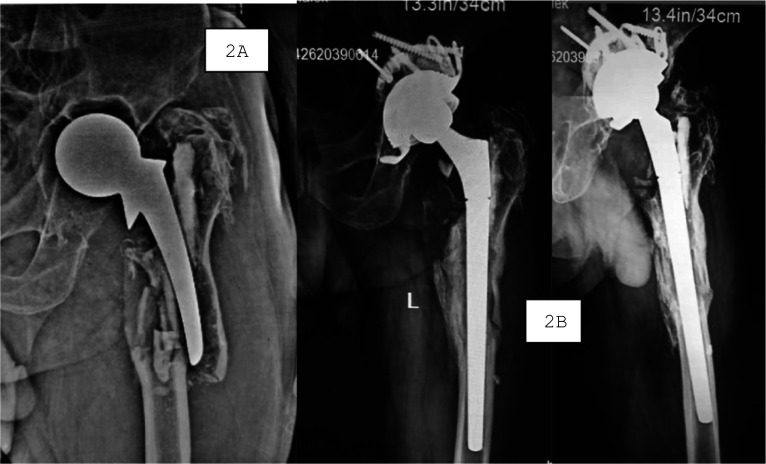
A 62-year-old male patient who underwent revision total hip arthroplasty using a modular cementless long stem (LIMA^™^) for a periprosthetic femoral fracture. A AP radiograph of the left hip and femur showing the fracture pattern prior to revision. B Final follow-up AP radiograph demonstrating stable fixation, satisfactory alignment, and successful integration of the modular long stem
